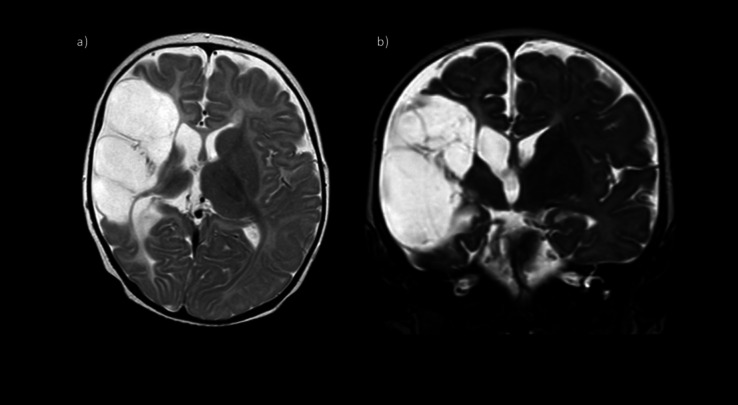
Axial (a) and coronal (b) T2-weighted images showing an extensive area of encephalomalacia involving the right frontal, temporal, and parietal lobes consistent with an old right middle cerebral artery territory infarct
